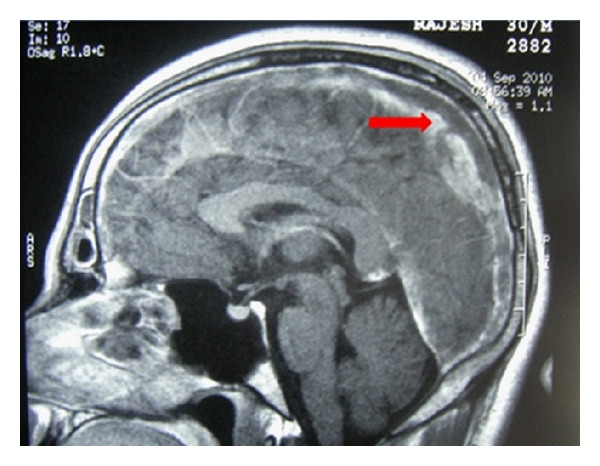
MRI scans showing cortical venous thrombosis.
